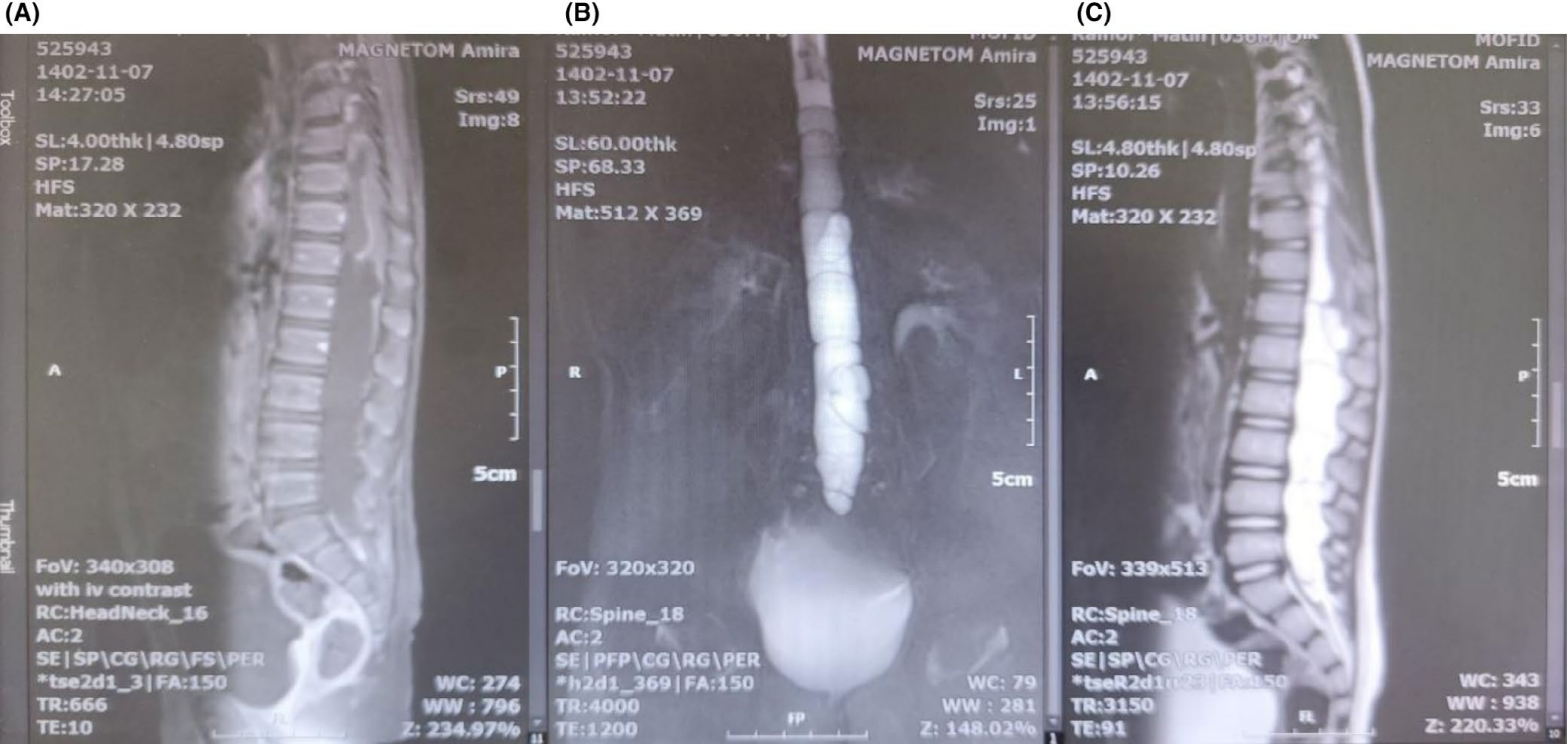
Preoperative thoracolumbar imaging. (A) T1‐weighted MRI with gadolinium injection (left), (B) Myelogram (middle), (C) T2‐weighted MRI with gadolinium injection (right). They depict well‐defined large hetrosignal multicystic lesions extramedullary intradural extending from T8 to S2 that caused compression and posterior displacement of cord.
